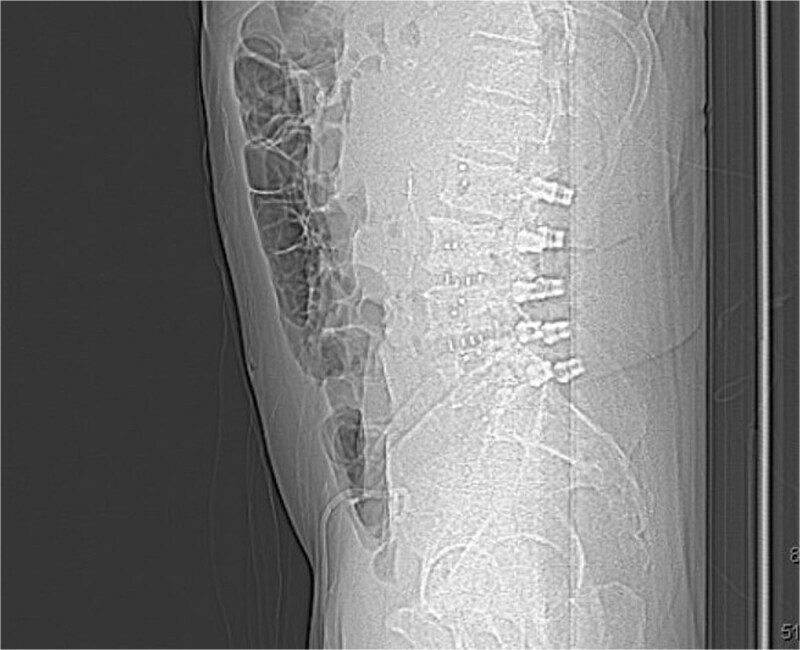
Postoperative sagittal X-ray demonstrating a carbon fiber plate and screws (lateral view).
